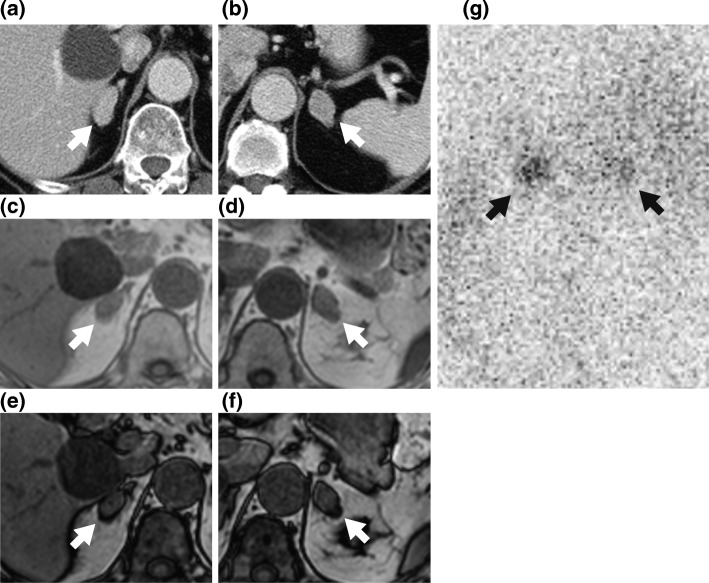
Imaging study of bilateral adrenal masses. Computed tomography scan shows bilateral adrenal tumors. (a) right side, 25 × 13 mm and (b) left side, 18 × 15 mm. Magnetic resonance imaging showed low intensity loci in adrenal tumors in “out phase” compared with “in phase” of chemical shift imaging on T1-weighted image. (c) right, in-phase; (d) right, out-phase; (e) left, in-phase; (f) left, out-phase. (g) ^131^I-adosterol scintigraphy shows bilateral adrenal accumulation. Arrows in each image designate the adrenal tumors or more accurately where the adosterol is accumulating in the adrenal tumors
